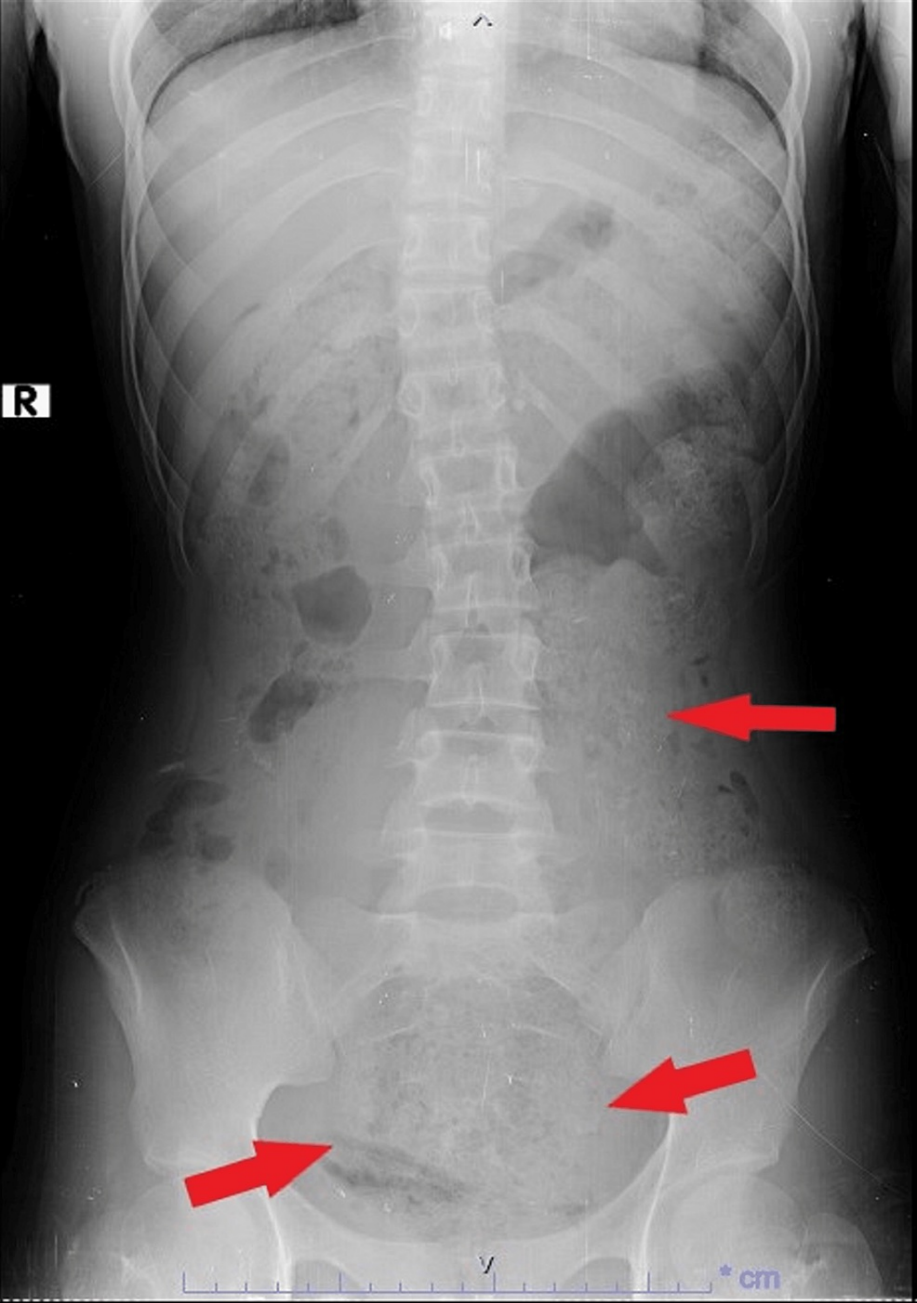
The patient's abdominal X-ray Red arrows reveal a loaded colon and an apparently loaded rectum.
